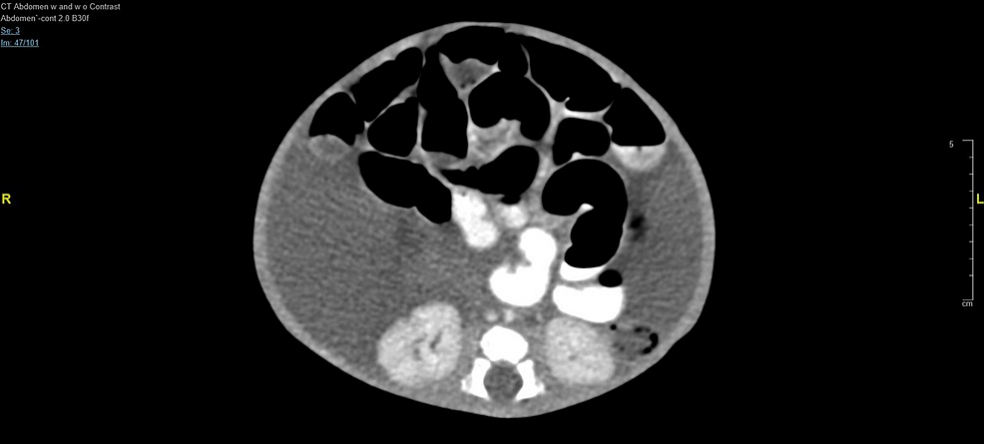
Computed tomography axial view at the level of lumber spine, showed marked abdominopelvic ascites.
